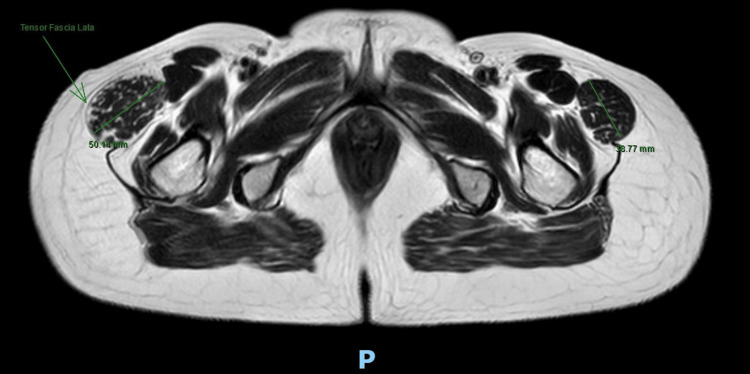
T1-Weighted MRI: Axial cut Hypertrophied tensor fasciae latae muscle (TFLM) (arrow), and transverse measurements of both TFLM (50.14 mm Right and 38.77 mm Left).
